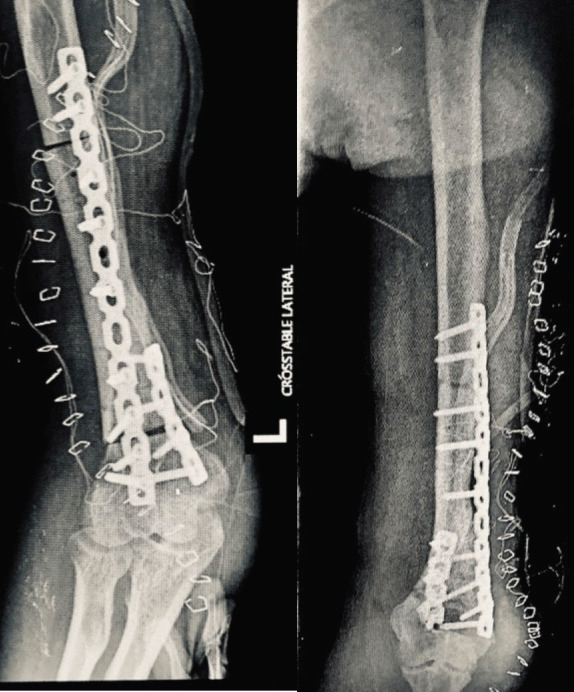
Postoperative (a): anteroposterior and (b): oblique radiographs of the left arm showing stable reconstruction of the humerus with acceptable alignment.
